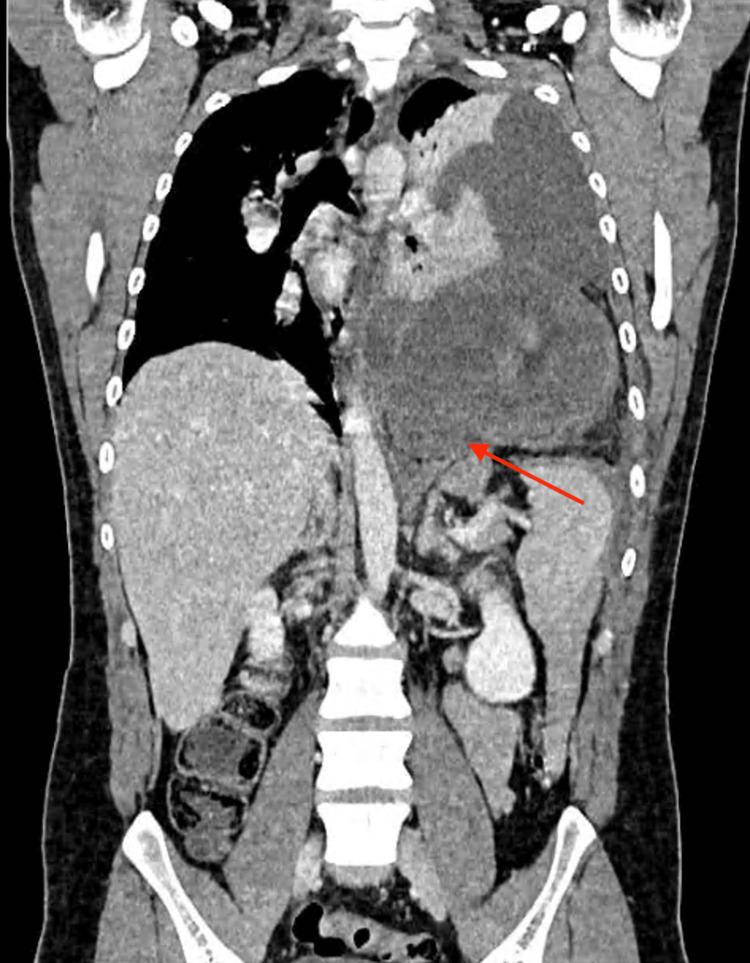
Coronal contrast-enhanced CT scan demonstrating a left-sided diaphragmatic defect (arrow) with herniation of the stomach into the thoracic cavity, associated with lung compression.
